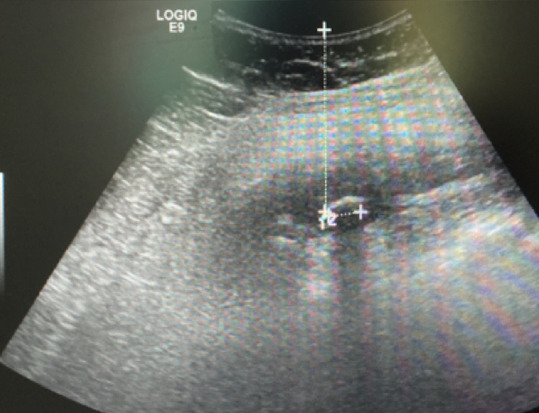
ultrasound images of the lost gallstone
